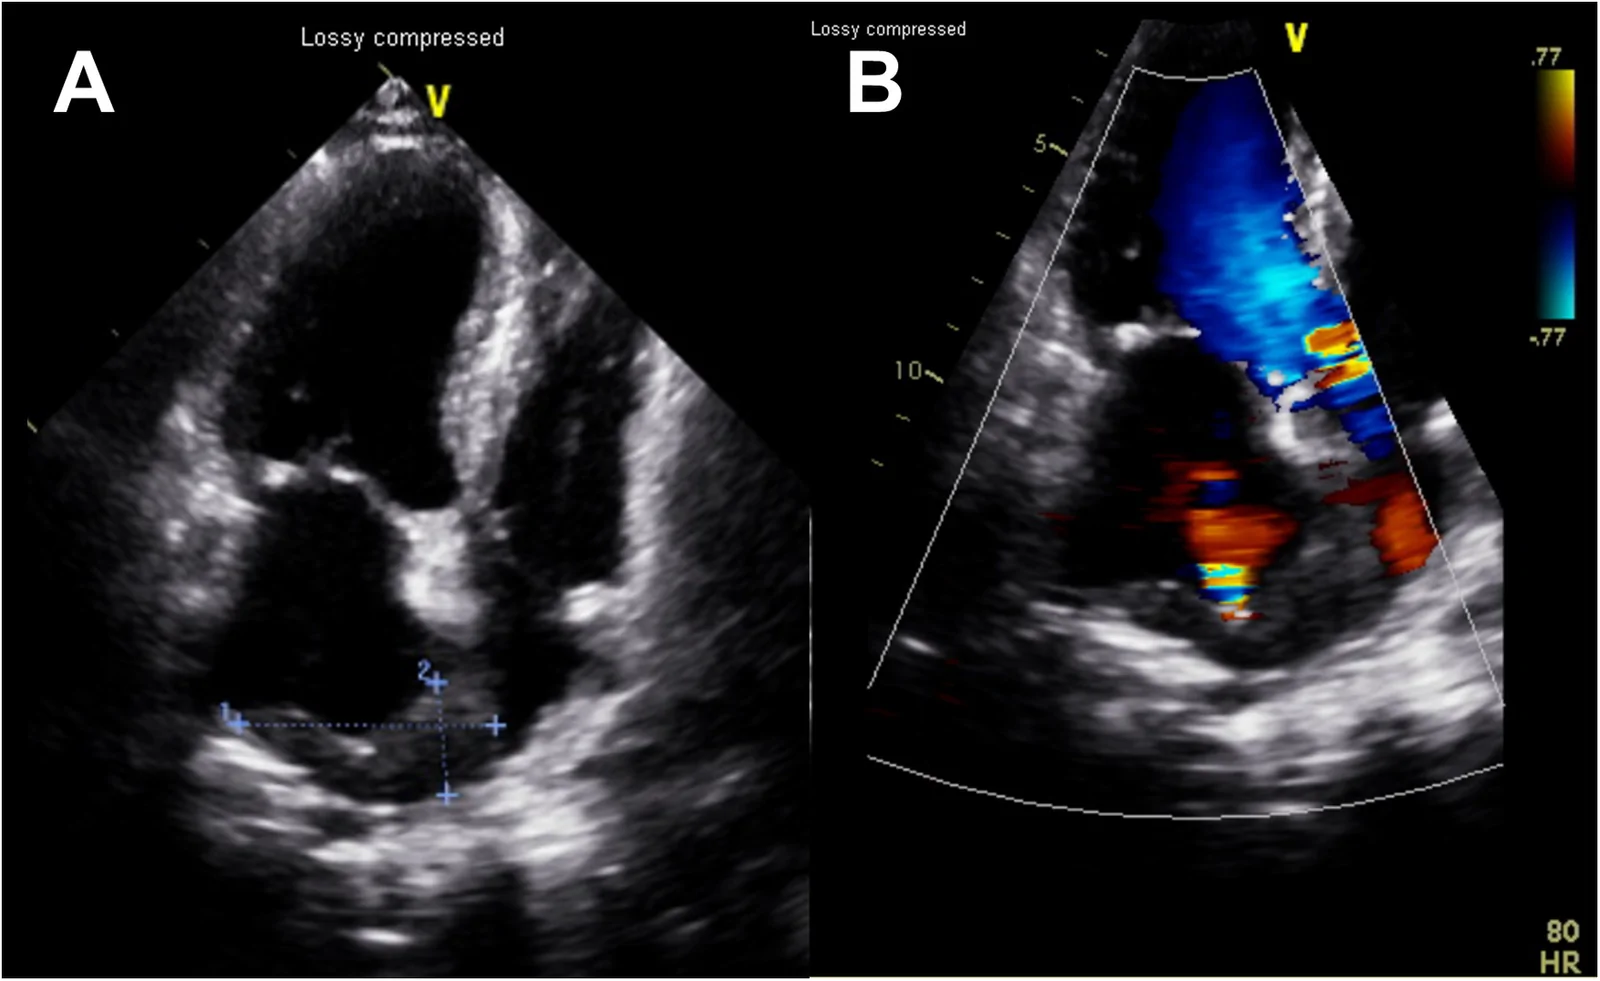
(A) Transthoracic echocardiography apical four chamber view showing broad mass (22.4 × 51.0 mm) with moderate echogenicity at posterior wall of the left atrium. (B) Color Doppler demonstrating an inflow jet from right pulmonary vein.
